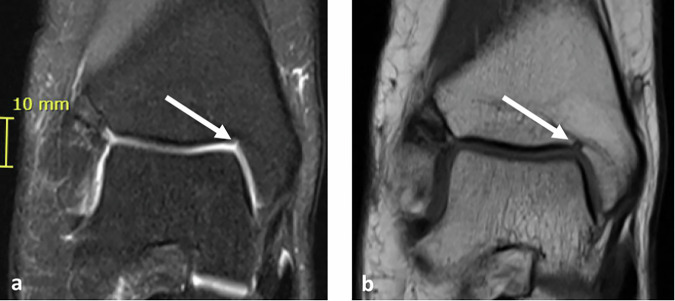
MRI of the right ankle in a 16-year-old female with a small and shallow type 1 NOH (white arrows). Dimensions of the notch are 2 mm (height) 2 mm (width) and 4 mm (depth). a Proton density weighted with fat suppression and (b) T1 weighted coronal sections
